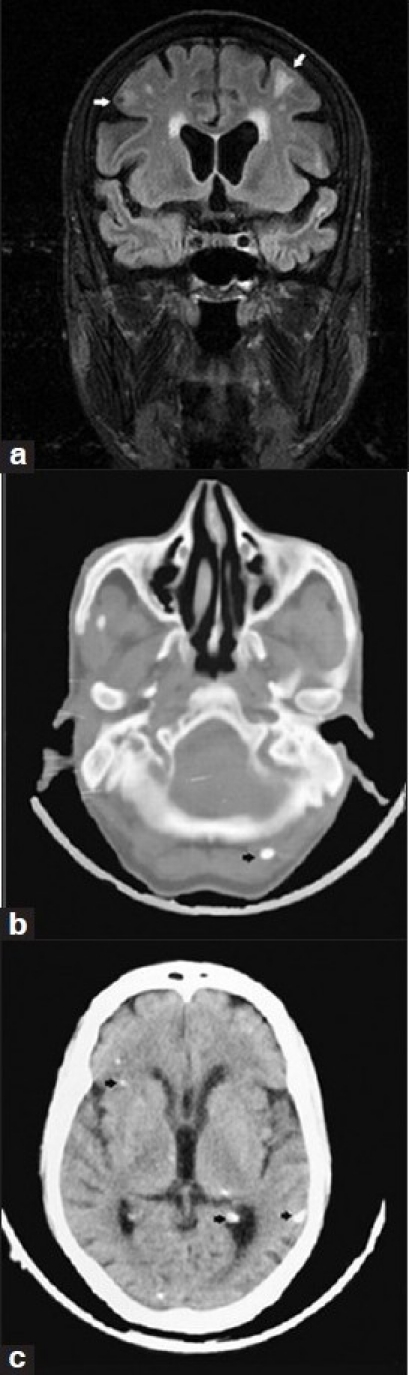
(a) Magnetic resonance imaging of the brain showing a hypodense lesion surrounded by perilesional edema in the right parietal lobe and edema in the left parietal lobe, (b) The computerised tomography scan of the brain showing a calcified lesion in the soft tissue of the scalp and (c) multiple calcified lesions located bilaterally in the brain parenchyma
